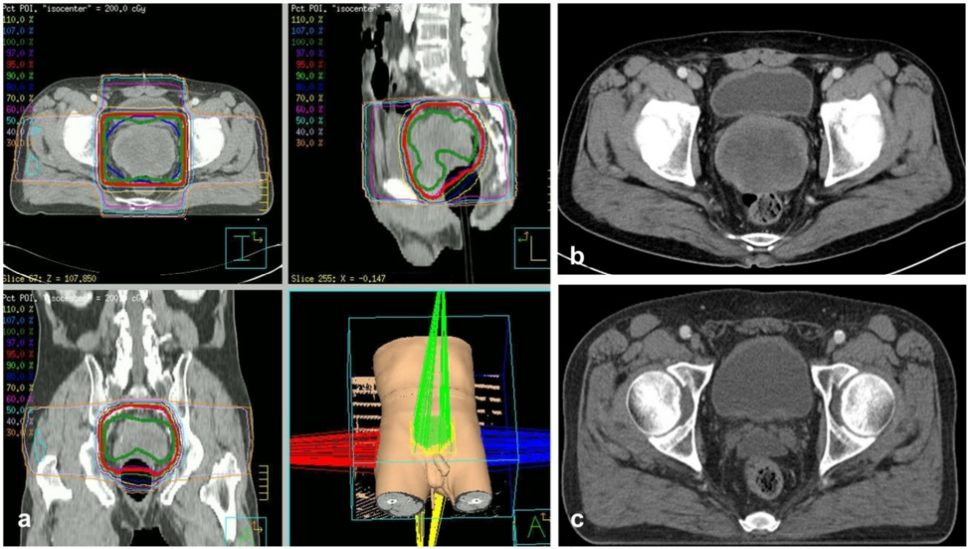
The three-dimensional radiotherapy for the rectal-shelf mass (a) Dose distribution for the three-dimensional radiotherapy plan for the metastatic myxoid liposarcoma, with a maximum size of 9.1 cm in the rectovesical pouch. The patient underwent salvage radiotherapy at a dose of 40 Gy delivered in 20 fractions of 2 Gy over 4 weeks between September and October 2012. (b) A computed tomography scan, obtained in November 2012 after completion of radiotherapy, showed that the metastatic mass had decreased by 6.9 cm in size. (c) A computed tomography scan, obtained in May 2013, showed that the mass had decreased by 4.0 cm in size.
